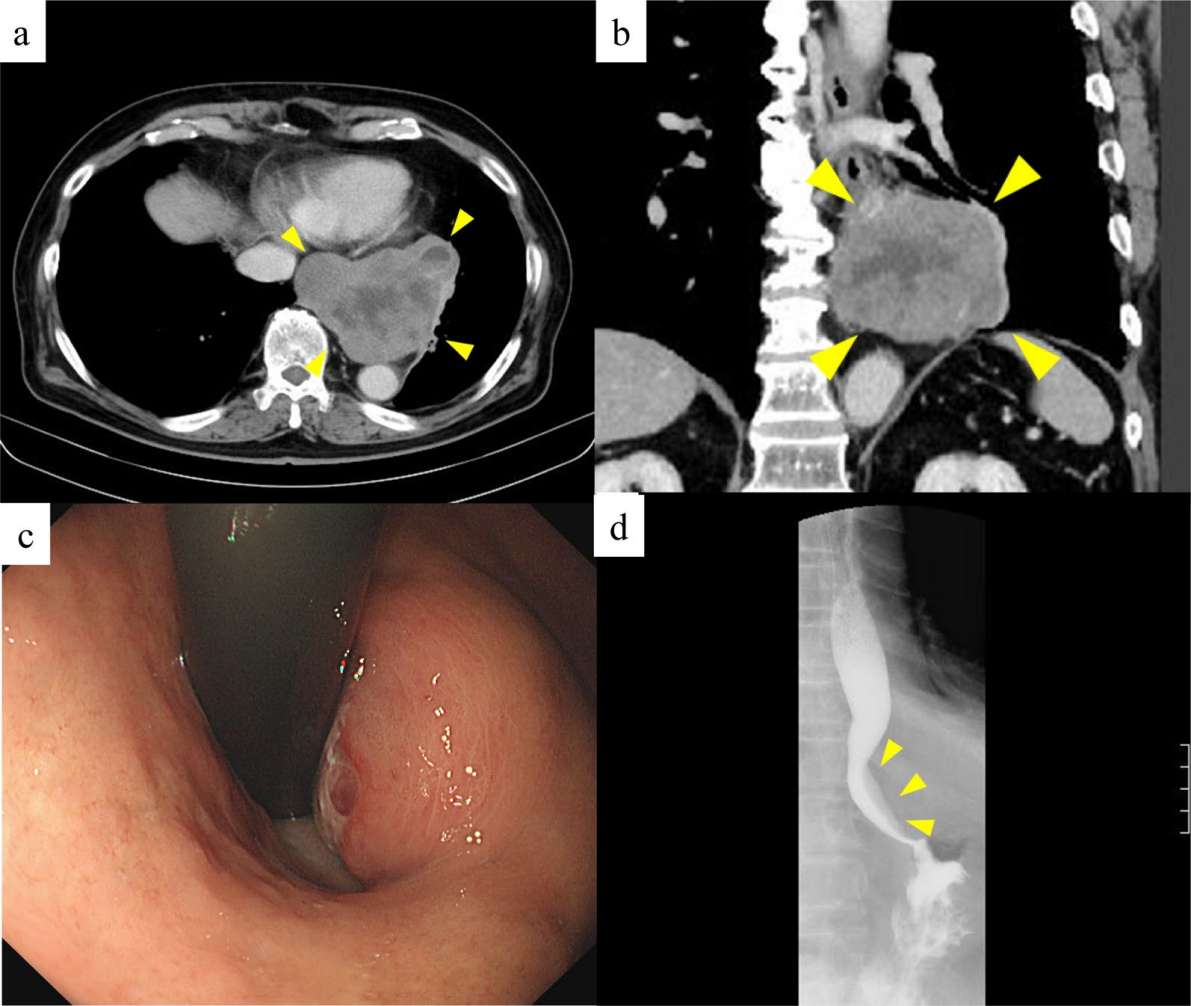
CT imaging before neoadjuvant therapy with imatinib shows that the tumor size is about 100 mm in diameter (a, b). Esophagogastroduodenoscopy reveals a submucosal tumor with a narrowed lumen and ulceration in the lower esophagus (c). Upper gastrointestinal series reveals compression towards the left side of the esophagus without mucosal changes (d)
